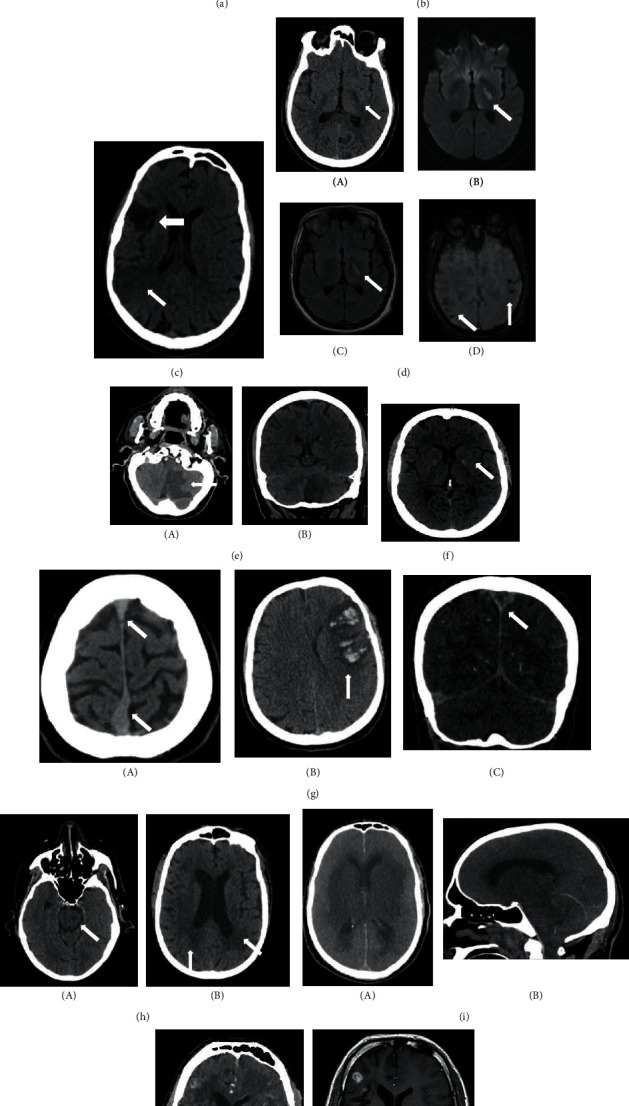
Brain imaging showing brain abnormalities in 10 patients (a–j). (a) Noncontrast CT head showing minor periventricular hypodensity suggestive of small vessel-related ischemia. (b) Noncontrast CT head showing multifocal white matter hyperdense foci suggestive of petechial haemorrhages (arrows pointing to areas of haemorrhage). (c) Noncontrast CT head showing old (big arrow) and evolving/new (small arrow) right MCA territory infracts. (d) (A) CT head showing low attenuation in the left internal capsule (B), (C) MRI showing left internal capsule high signal on diffusion weighted and FLAIR sequences, and (D) MRI gradient echo sequences showing blooming artifact suggesting blood break down products. (e) Axial and coronal non contrast CT head showing acute left cerebellar infract. (f) Noncontrast CT head showing a small area of low attenuation in the left internal capsule suggestive of a focal infract. (g) Noncontrast CT head showing (A) dense superior sagittal sinus (SSS) suggestive of venous sinus thrombosis, (B) haemorrhagic venous infract, and (C) CT venogram showing nonopacification of SSS (empty delta sign) due to venous sinus thrombosis. (h) Noncontrast CT head showing (A) lacunar infract left pons and (B) periventricular low attenuation changes in keeping with small vessel ischemia. (i) (A) Axial and sagittal noncontrast head CT showing marked sulcal effacement, extensive oedema, and loss of grey-white matter differentiation. (B) Sagittal section shows herniation of cerebellar tonsils. Appearances in keeping with severe cerebral oedema with coning. (j) Postcontrast CT (A) and MRI (B) of head showing focal enhancing lesion in the right frontal lobe.
